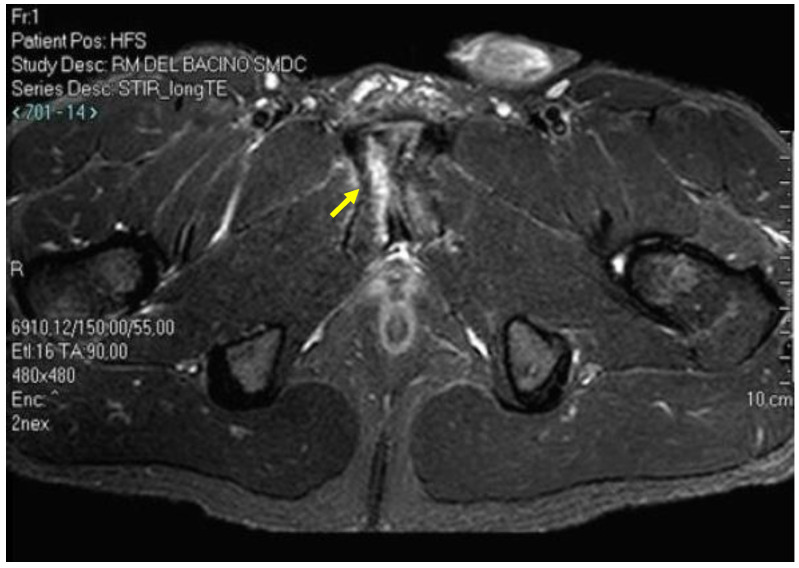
Axial STIR MRI showing a bone marrow oedema extending over the entire surface in an antero-posterior direction (arrow) of the right pubic ramus. Based on its extension, the bone marrow oedema is classified as Grade 3.
